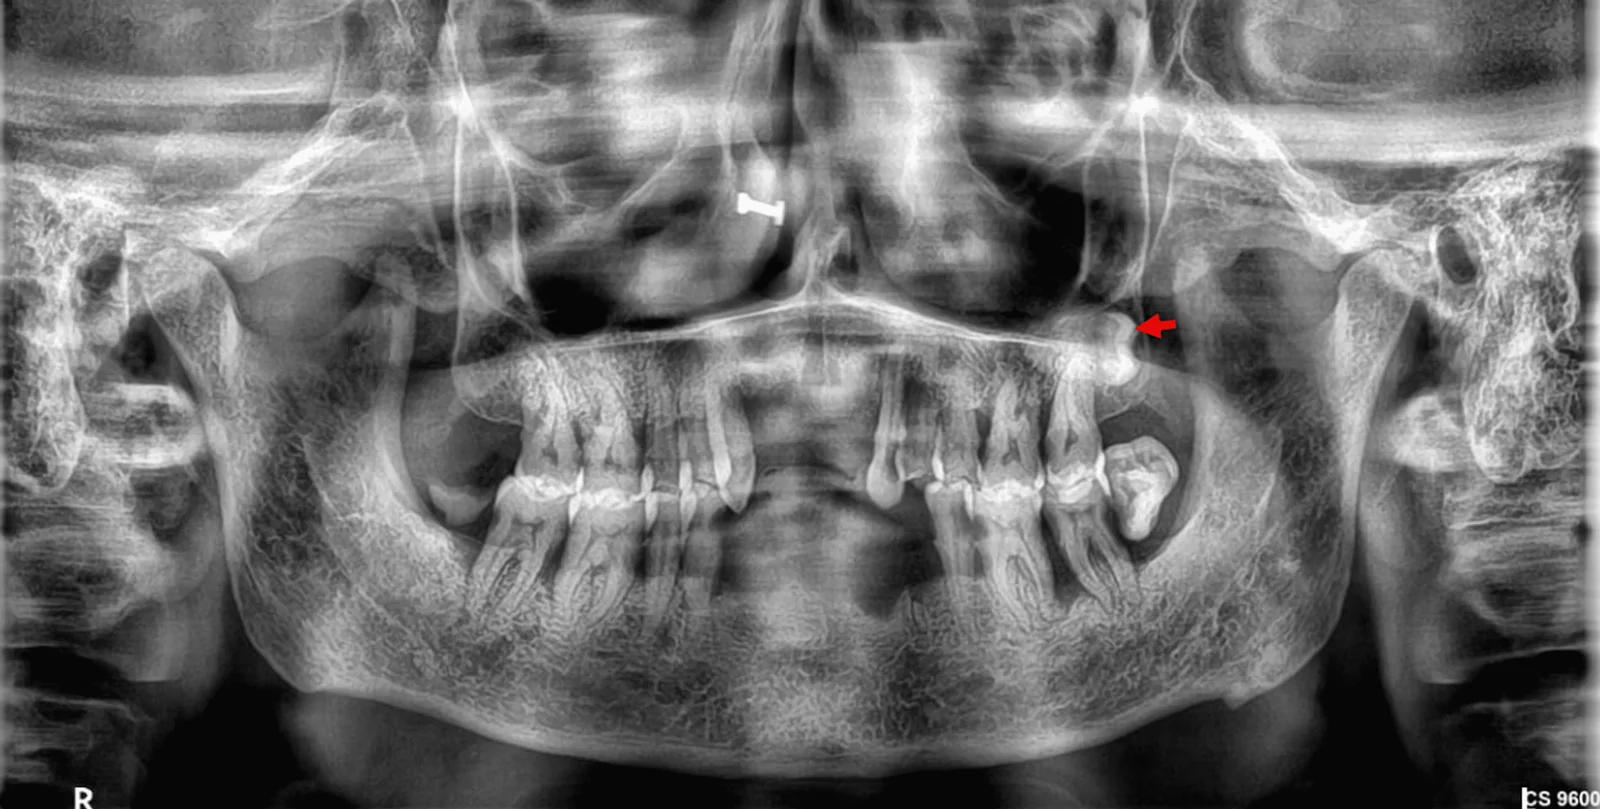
Digital orthopantomography revealed generalized alveolar bone loss and horizontal impacted left maxillary third molar (red arrow)
